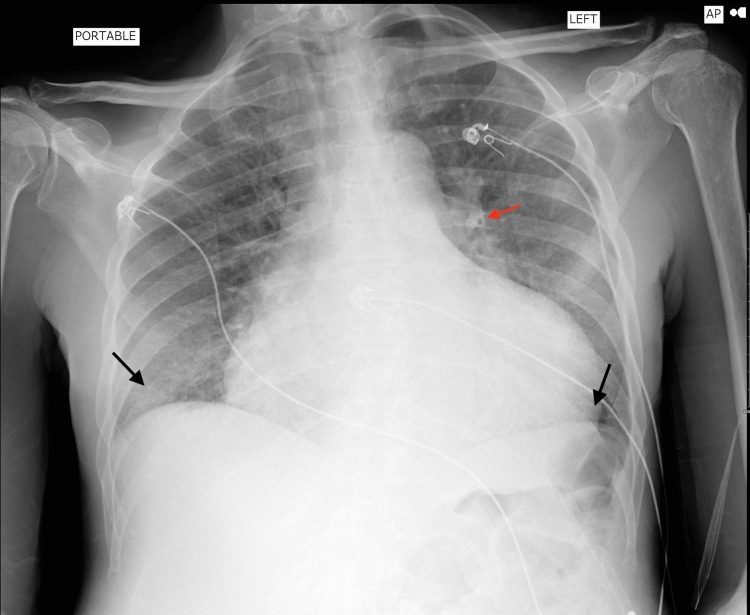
Anteriorposterior (AP) Chest X-ray: Black arrows point to regions of small bilateral pleural effusions without consolidations and the red arrow points to pulmonary vascular congestion.
